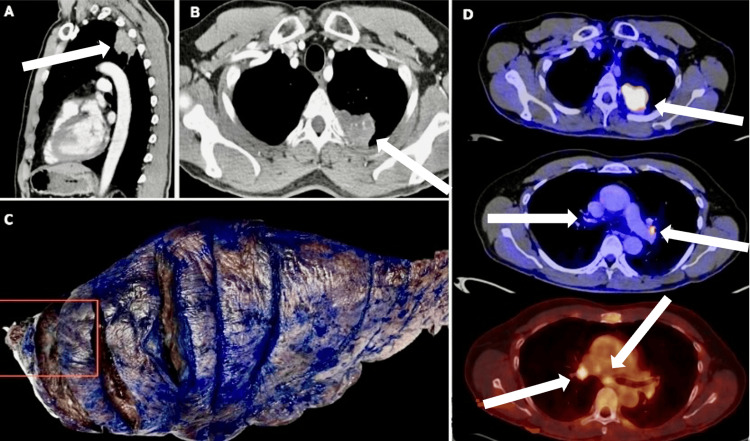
A, B: Contrast tomography of the thorax Mass with spiculated margins in the left upper lobe apicoposterior segment measuring approximately 42 x 32 x 45 millimeters with infiltration to the third rib. C: Lobectomy with costal bone condition. Rectangles indicate the thoracic wall. D: PET-CT: Pulmonary mass with SUVmax of 35.91 in contact with the major fissure and with the parietal pleura, with obliteration of the extrapleural fat, up to where the metabolic activity extends. Mediastinal adenopathies with an SUVmax of 7.45 are located in both the pulmonary hilar, paraaortic, and subcarinal regions.
